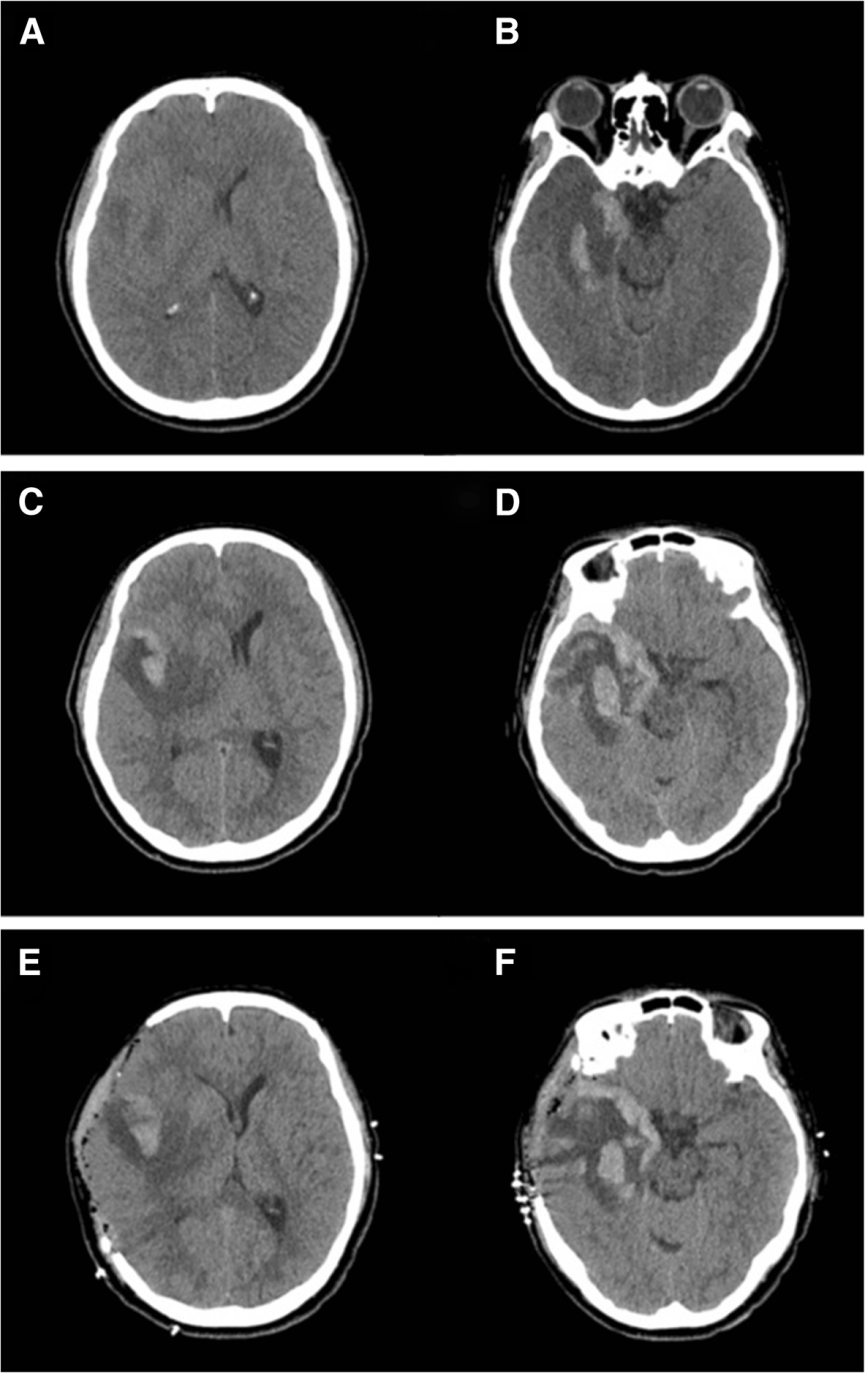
Serial follow up noncontrast brain CT. a, b: Brain CT taken on the day of admission to our institution (2017.12.19) shows a hemorrhagic transformation of the right temporal lobe lesion. c, d: Brain CT taken on day 3 of hospitalization (2017.12.22) shows an increased amount of temporal lobe hemorrhage and a leftward shift in the midline. e, f: Postoperative brain CT (2017.12.23) shows an improvement in midline shift with no significant increase of temporal lobe hemorrhage
